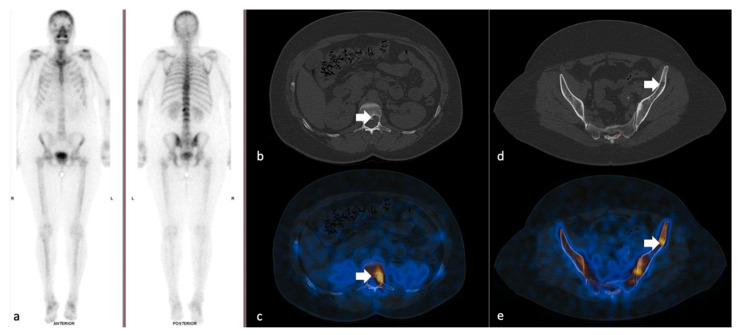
47 year-old female patient with breast carcinoma who underwent quantitative analysis SPECT-CT scanning: (a) whole body scan showing faint uptake in the L2 vertebral body; (b,c) high uptake in the L2 osteolytic lesion; and (d,e) osteolytic lesion on the iliac bone undefined on the planar scintigraphy showing increased radiotracer uptake.
